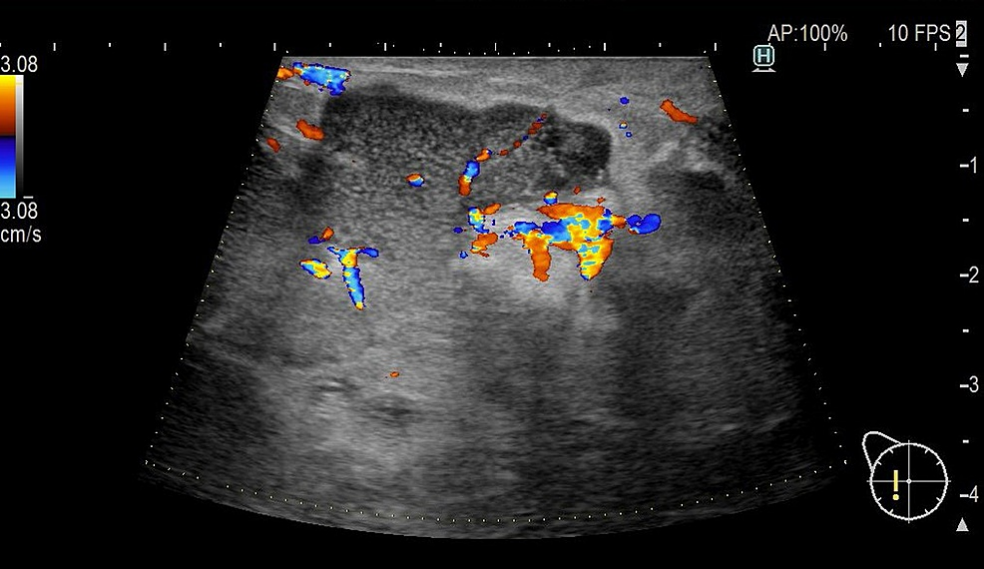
Color Doppler of the right breast
